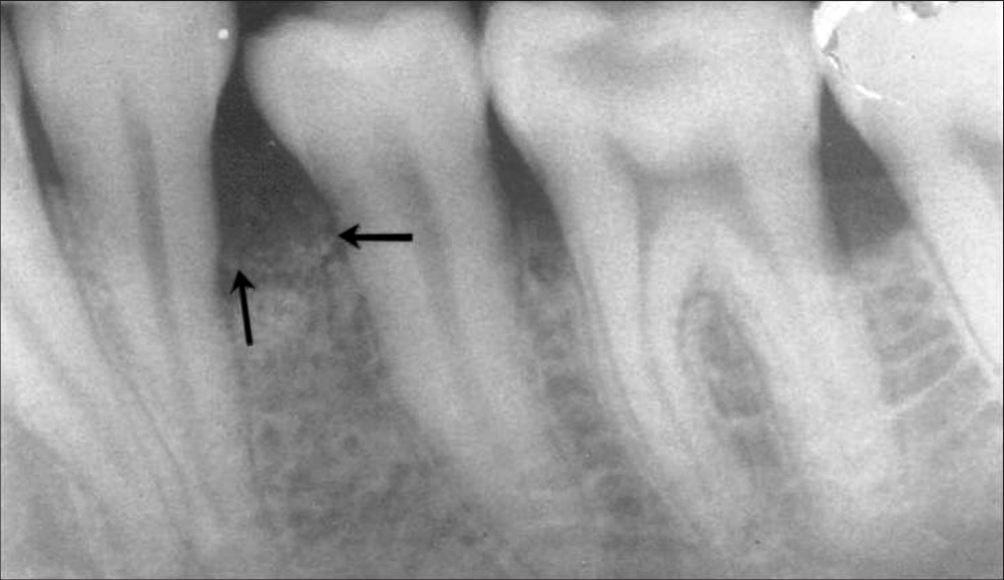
Nine month post treatment periapical radiograph depicts defect fill on the mesial aspect of tooth # 35
